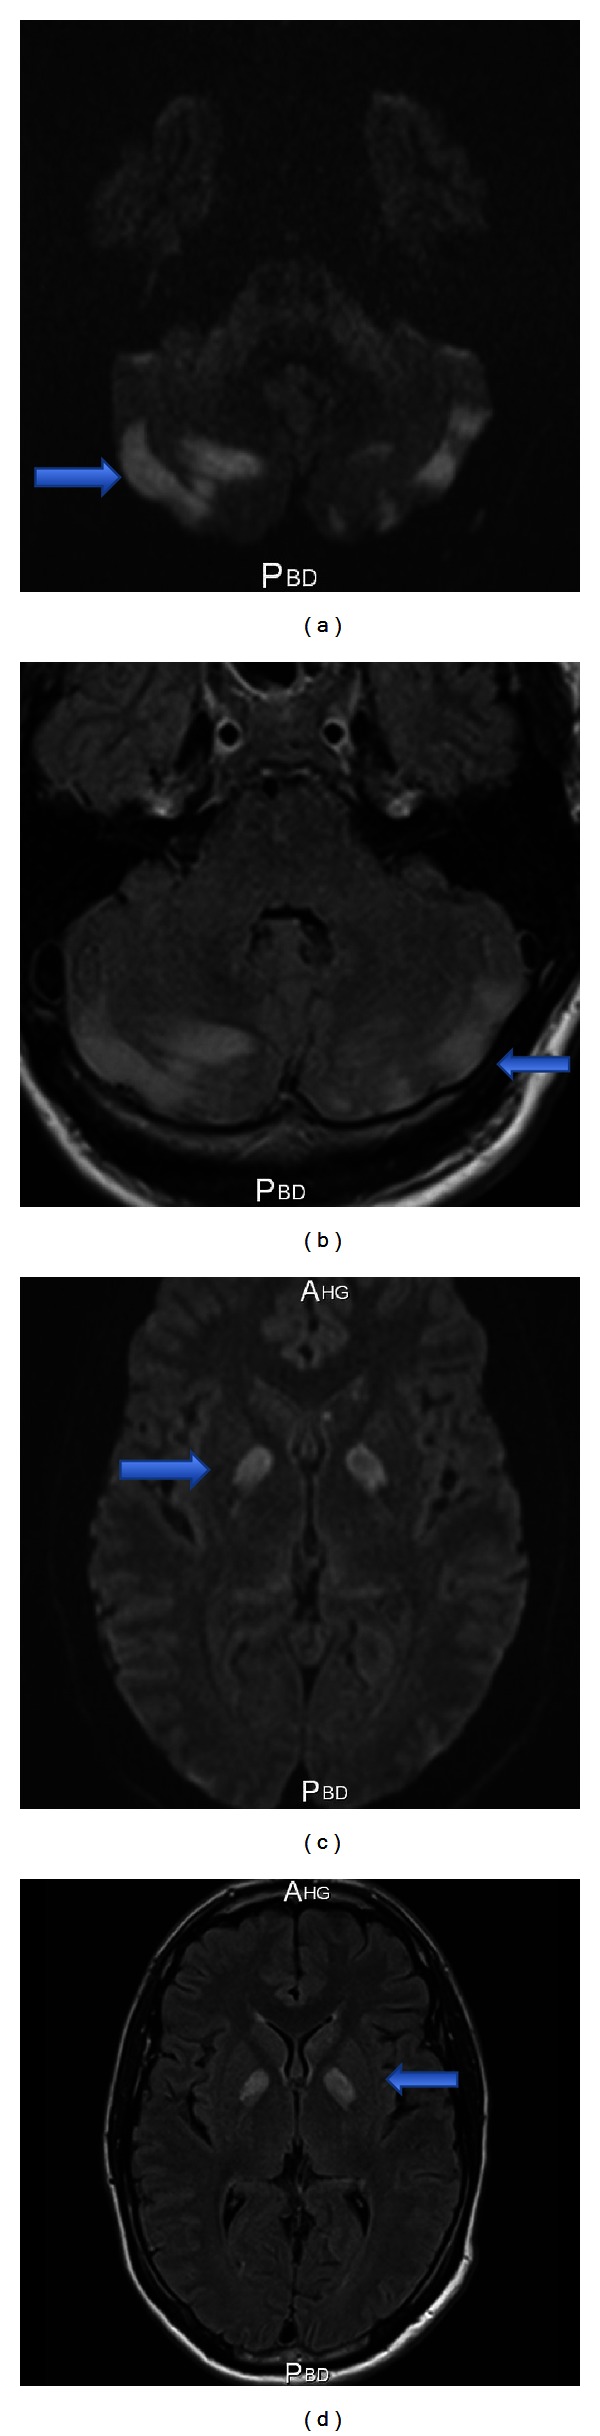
Cerebral IRM showing FLAIR (on the left side) and DWI (on the right) hyperintensities in both cerebellar hemispheres (panels (a)-(b)) and basal ganglia (panels (c)-(d)).
